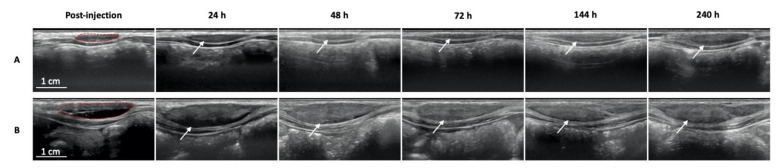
Longitudinal ultrasound imaging of (A) 0.2 mL and (B) 1 mL test item injection site over the course of the study. Images were acquired between 13 and 15 MHz along the sagittal plane, parallel to the needle insertion orientation. Bolus are highlighted in red post-injection and indicated by an arrow at later time points.
